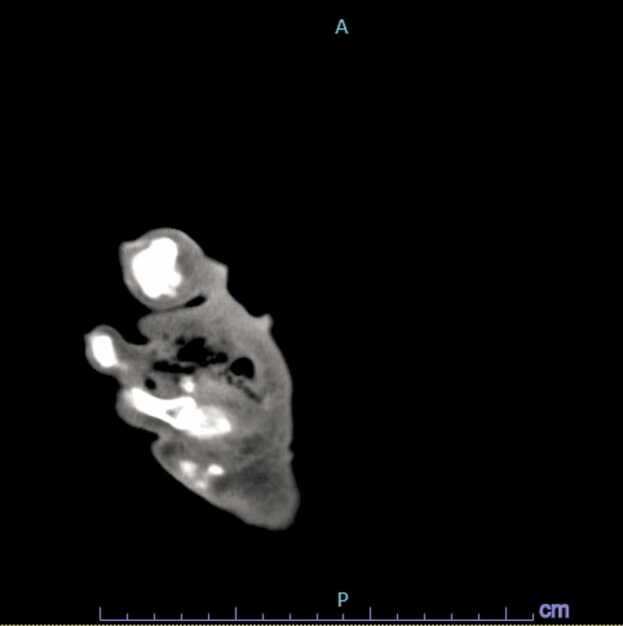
Computed tomography scan of the lower extremity showing subcutaneous gas on the right plantar surface.
